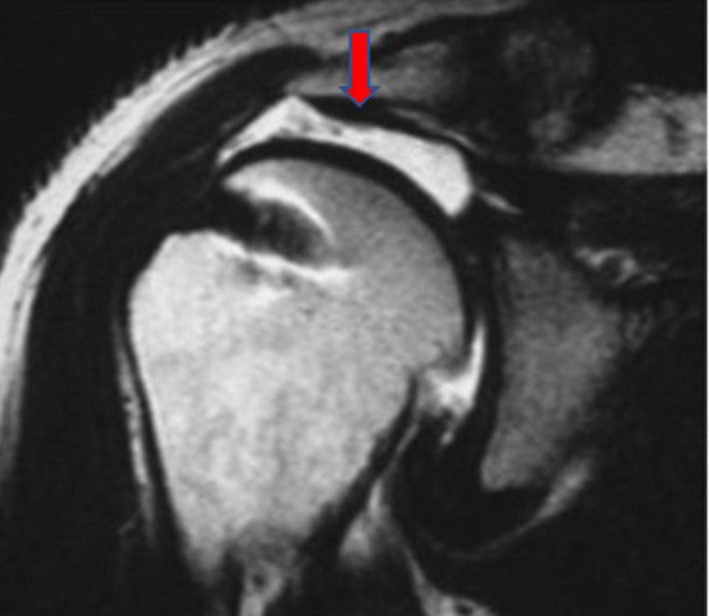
Example of MRI Sugaya type V of a non-enrolled patient
